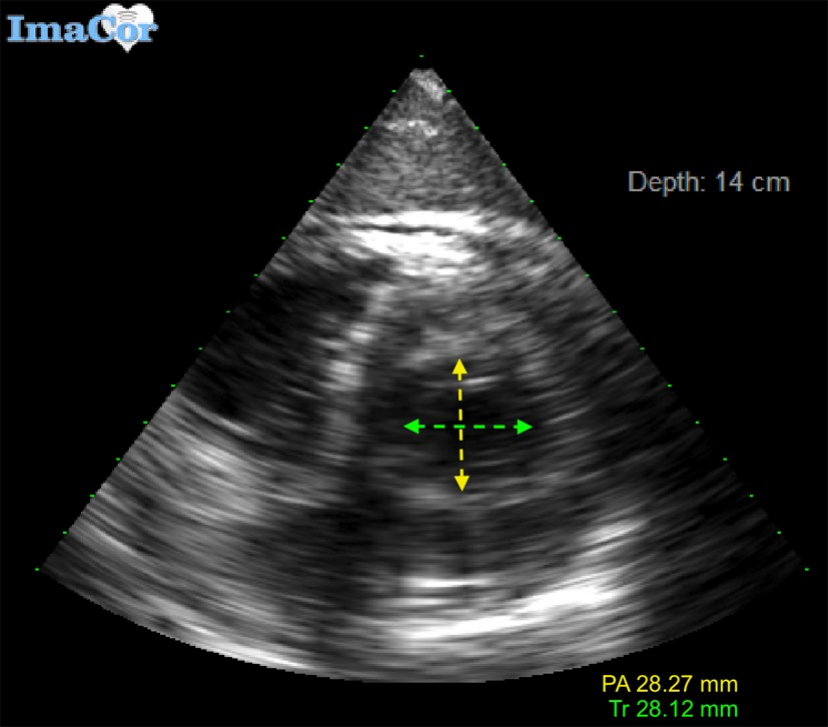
Transesophageal echocardiographic view demonstrating transverse and posteroanterior measurements of left ventricle in end-systole
